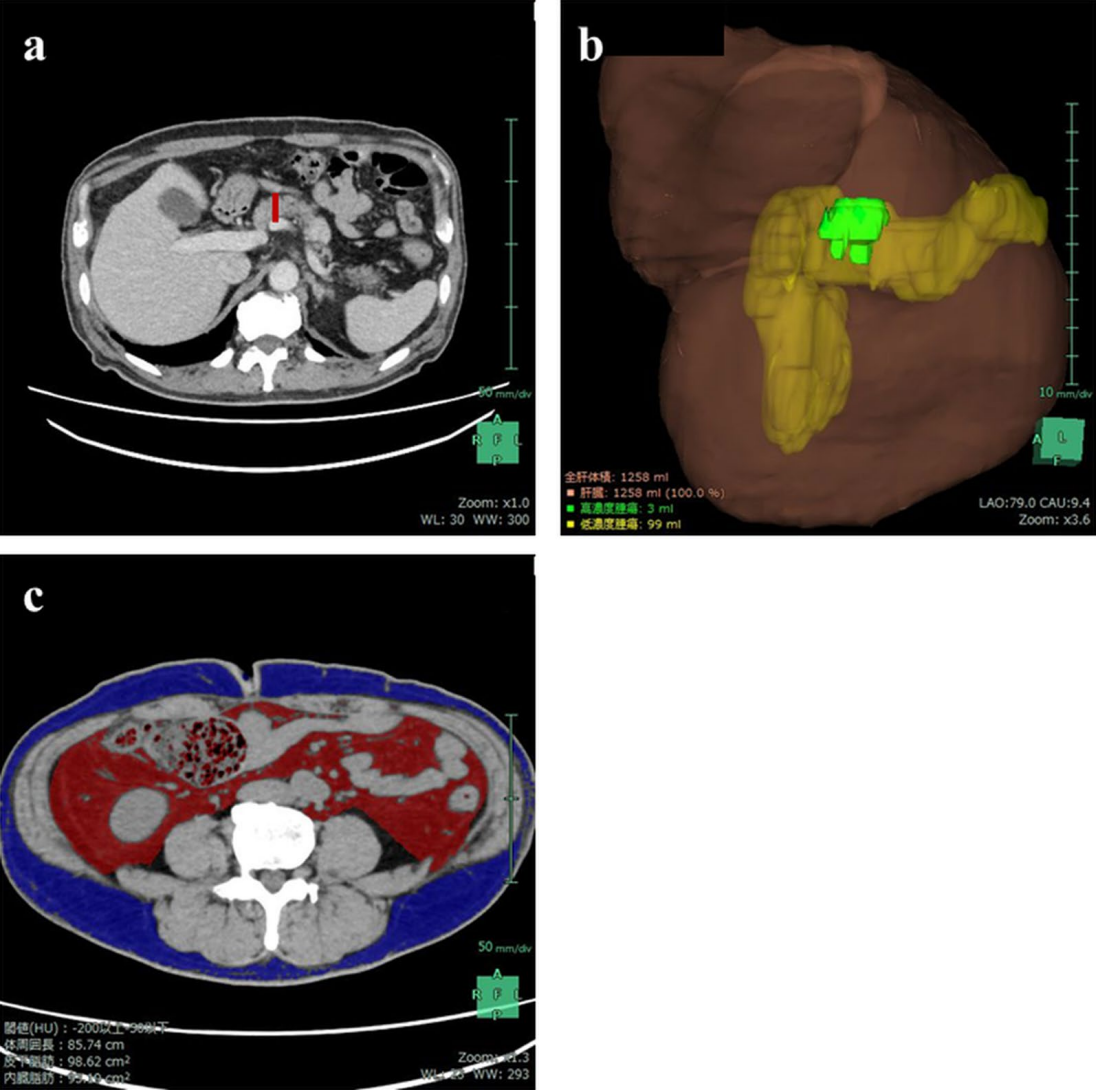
CT examination for evaluating LSG’s weight-loss and metabolic effects. a Pancreas parenchyma thickness decreased to 17 mm (red bar). b CT volumetry revealed that the PV also decreased 99 mL with no tumor growth. c Subcutaneous and visceral fat areas decreased to 98.6 cm^2^ and 93.2 cm^2^, respectively
